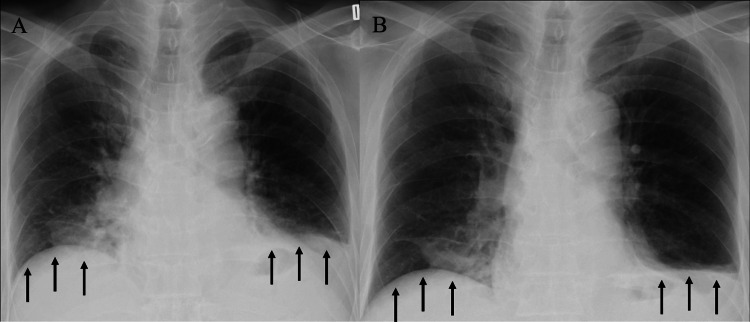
Chest radiographs during inspiration and expiration. Chest radiographs showed no appreciable difference in the diaphragm position between inspiration (A) and expiration (B), suggestive of bilateral diaphragmatic paralysis. The black arrow points to the diaphragm, which has poor movement during respiration.
